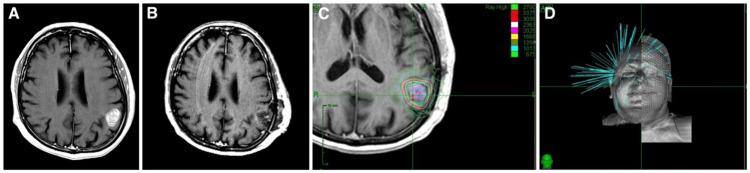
Examples of (A) MRI of brain metastasis prior to resection; (B) MRI of tumor bed after resection; (C) The 24 Gy/3 fraction dosimetry for stereotactic radiosurgery; and (D) CyberKnife beam arrangement
